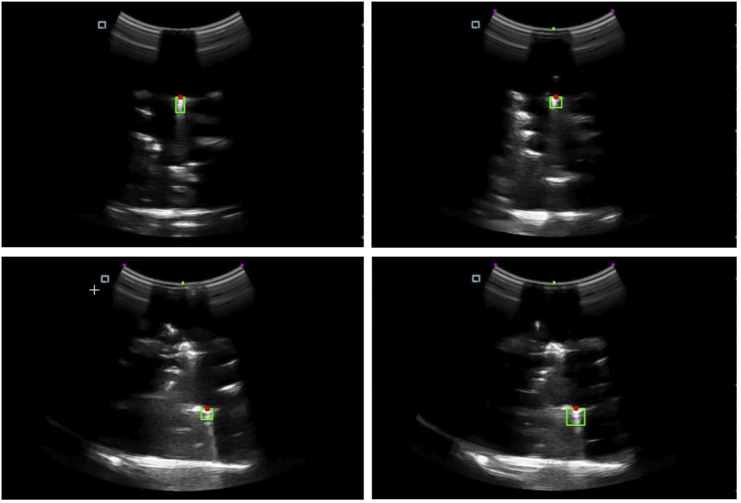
The needle tip localization in the ultrasound image. The green area indicates the region containing the needle tip, while the red dot marks the detected needle tip position.
